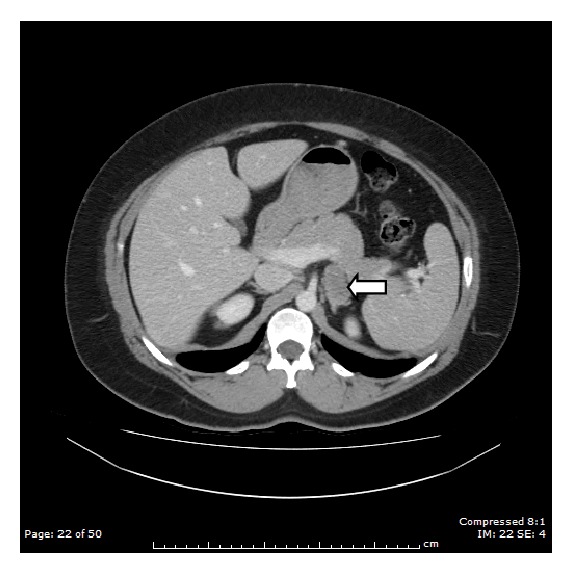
A CT scan of the abdomen showing a well encapsulated “adrenal” mass (solid white arrow).
